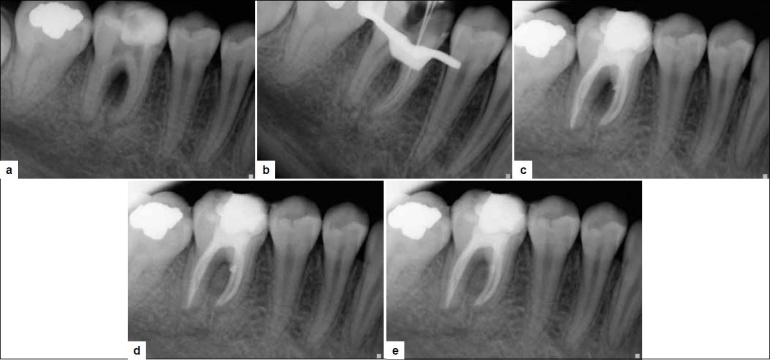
a) Pre-operative radiograph showing large furcal radiolucency and apical radiolucency at the mesial and distal roots. b) Mesiobuccal and mesiolingual canal location with no. 15K-file. c) MTA condensed into the perforation site; slight extrusion of MTA near distal aspect. d) 6 months follow up, decreased radiolucency and osseous healing at the furcation. e) 2 years follow-up
